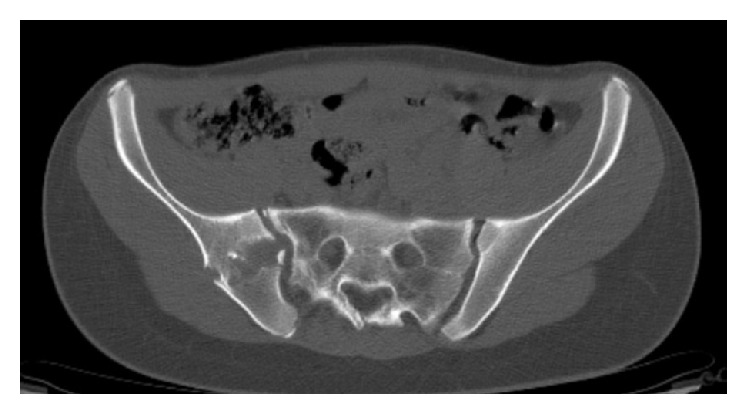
Computed tomography shows erosions of the right ilium, involving the SI joint.
